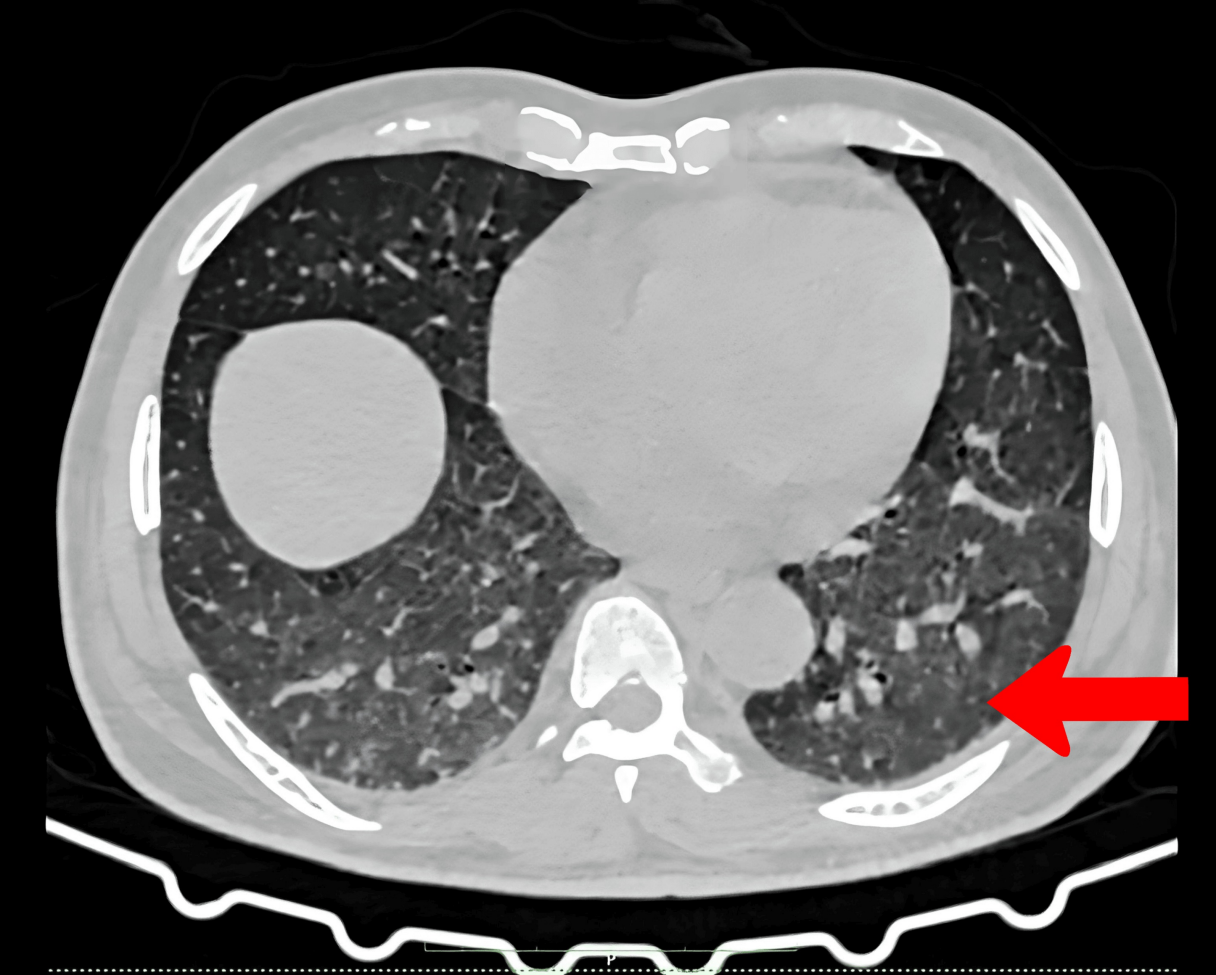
High-resolution CT images of the axial section of the thorax The red arrow shows ground-glass opacities in bilateral lung fields.
